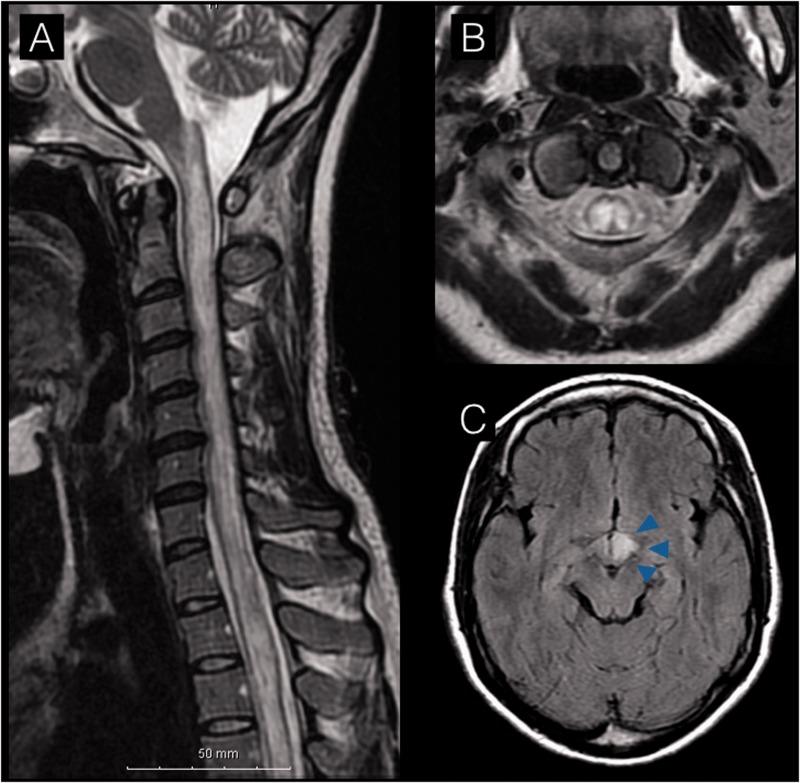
(a) Spinal magnetic resonance imaging on day 2 exhibited a T2-hyperintense lesion extending from the medulla oblongata to the thoracic cord. (b) Axial image at the C1 level revealed an extensive lesion, mainly involving the central gray matter. (c) Axial image at the midbrain level showed a left hypothalamic lesion. (a) and (b): T2-weighted images. (c): Fluid-attenuated inversion recovery image.
